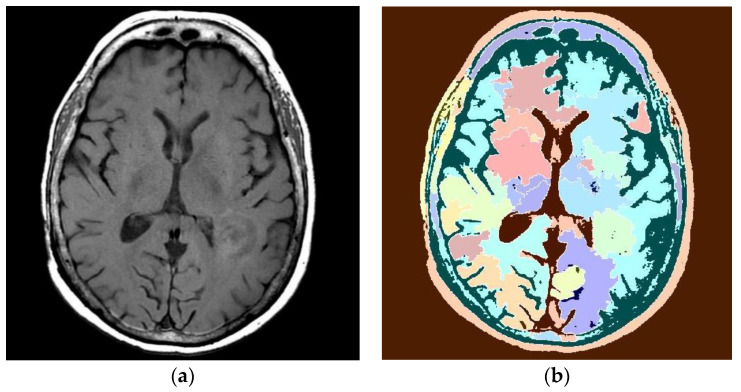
MRI of a tumor. (a) Original MRI. (b) Area recognition with original magnetic resonance image.
